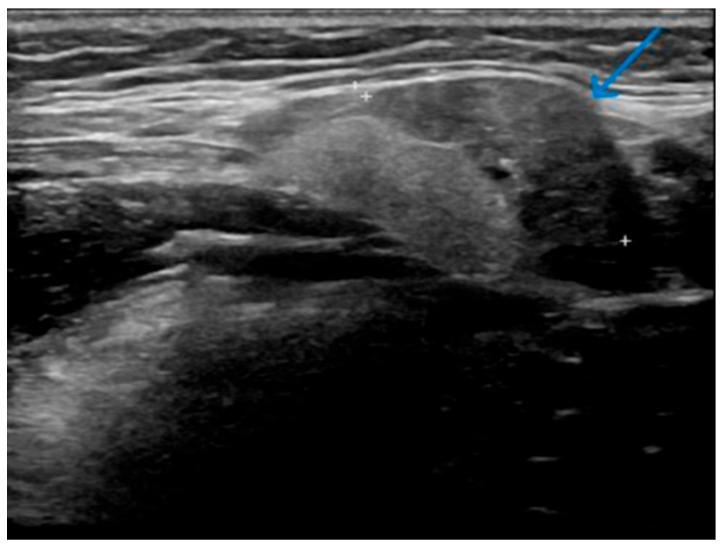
Patient Z—Initial ultrasound scan of affected SMG. Well-defined, focal hypoechoic area in the left SMG (indicated by the blue arrow) with mild focal duct dilatation. The "+" markers represent measurement callipers.
